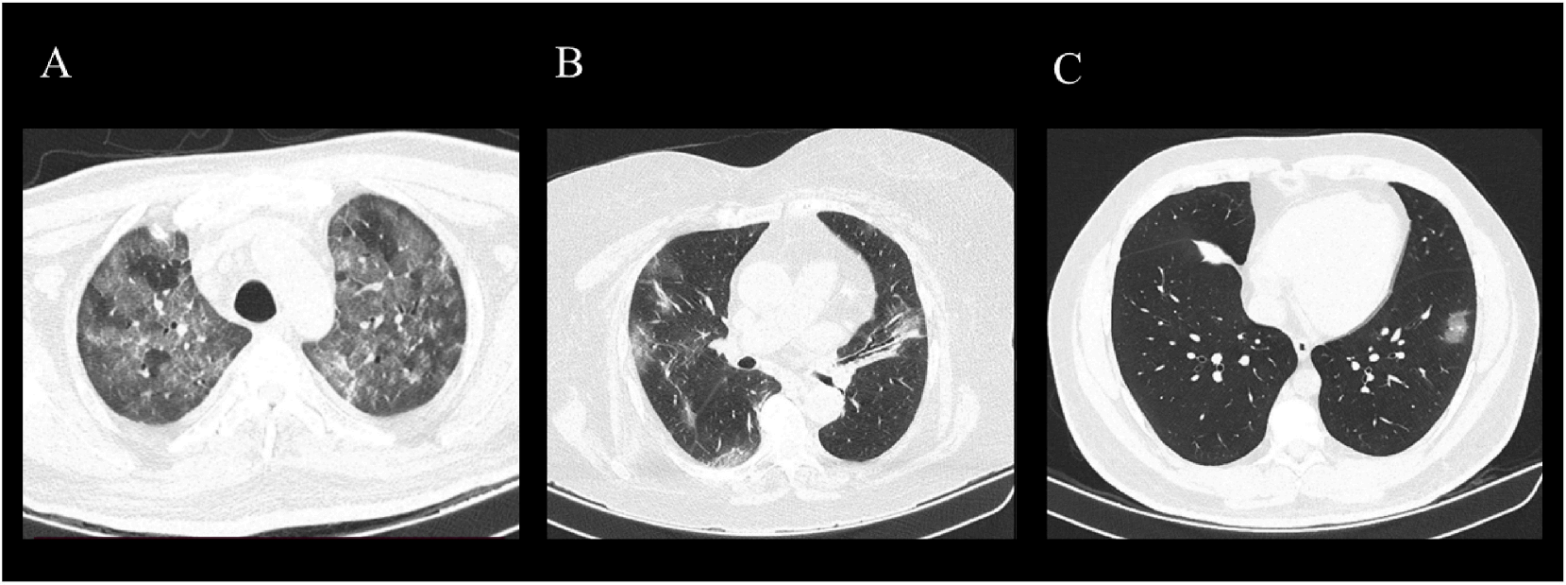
Tomographic findings A) Computed tomography, axial cut of the lung. Severe affection of the lung, both superior lung lobules are seen with a diffuse ground-glass image with air entrapment. Interlobular septal thickening, as well as vascular thickening pattern. B) Computed tomography, axial cut of the lung. Moderate affection of the lung, ground-glass zones with subpleural distribution are observed, with interlobular septal thickening and vascular thickening. C) Computed tomography, axial cut of the lung. Mild affection of the lung, small ground-glass opacities in the lower left lobule are observed.
